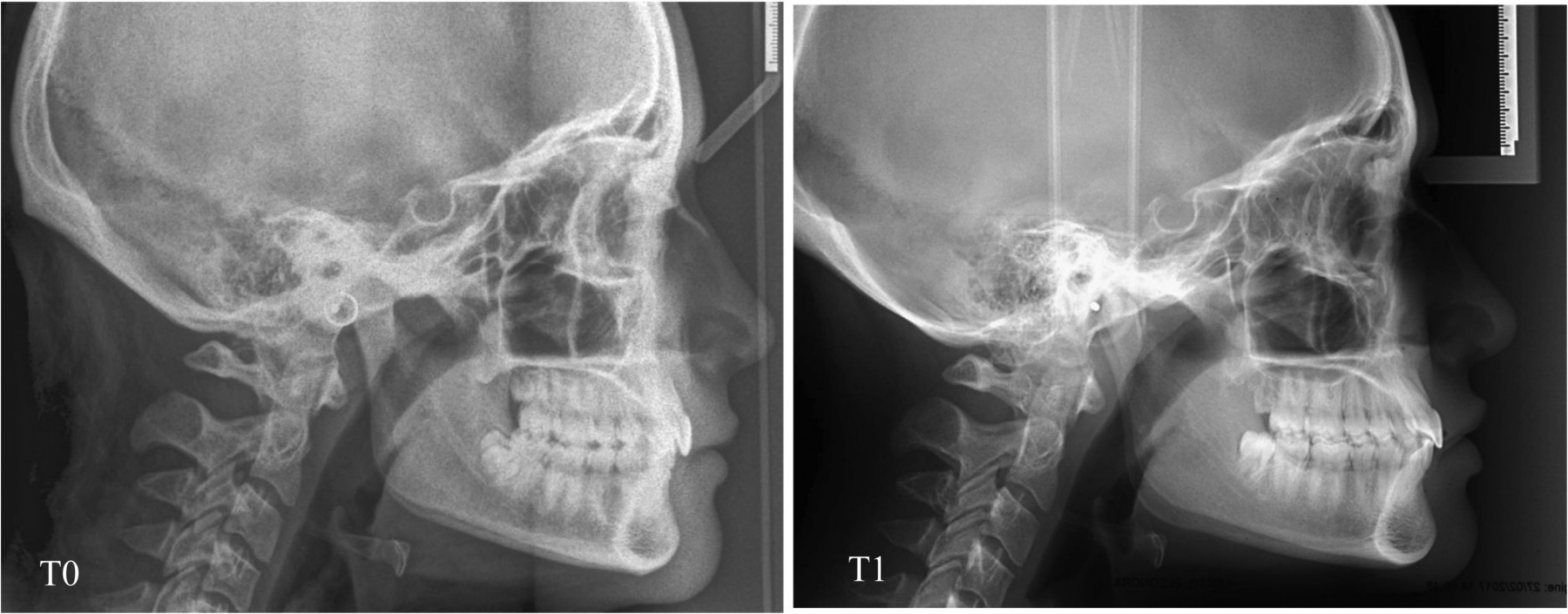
Lateral cephalometric radiography at time T0 and T1 of a patient in the MB.
